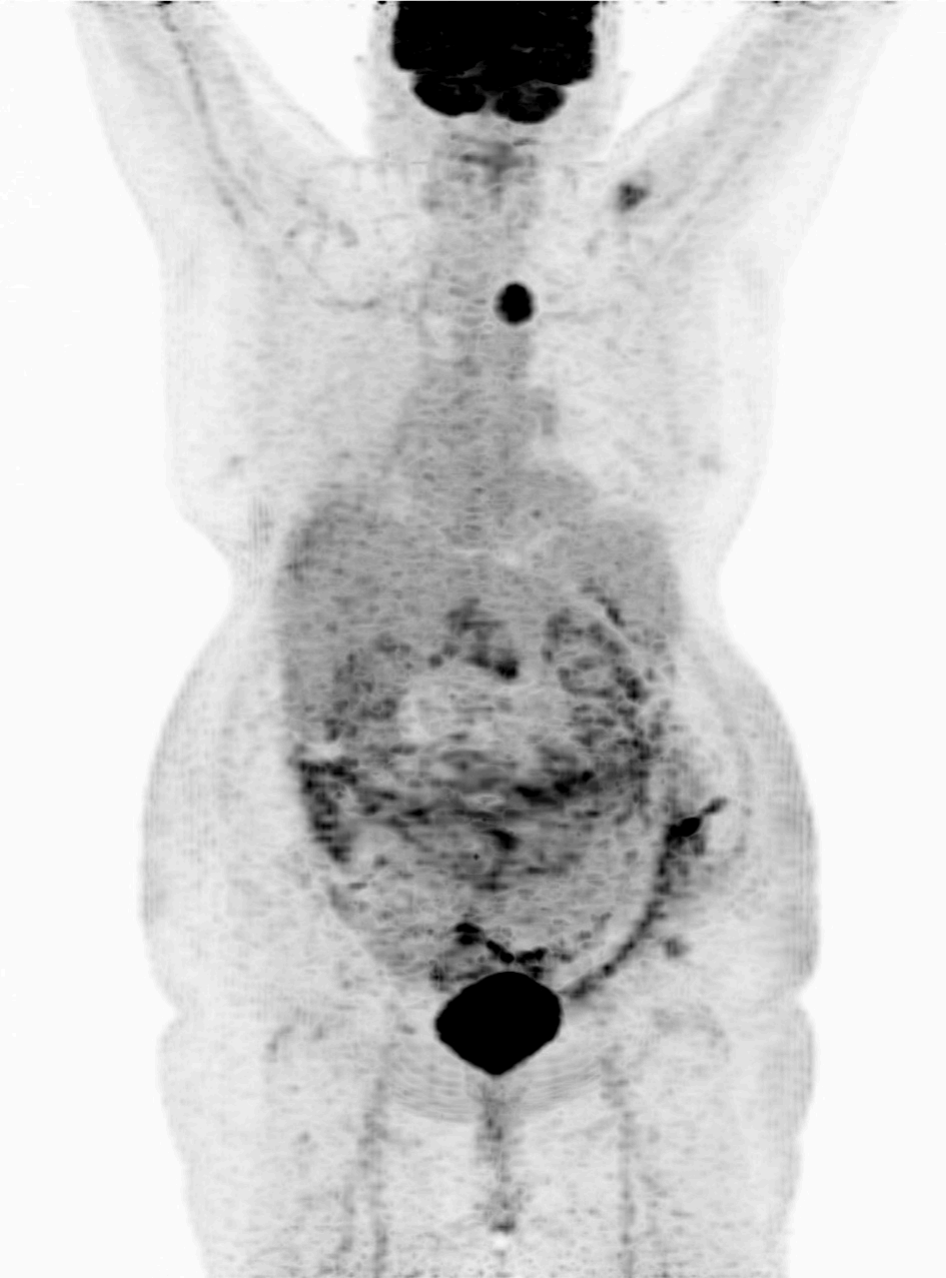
Maximal intensity projection of our patient. Acquisition of broadcoast images realized 60 min after injection of 252 Megabecquerels of FDG-IBA in a vein of the right wrist. Visualization of the supra-diaphragmatic isolated hypermetabolism.
